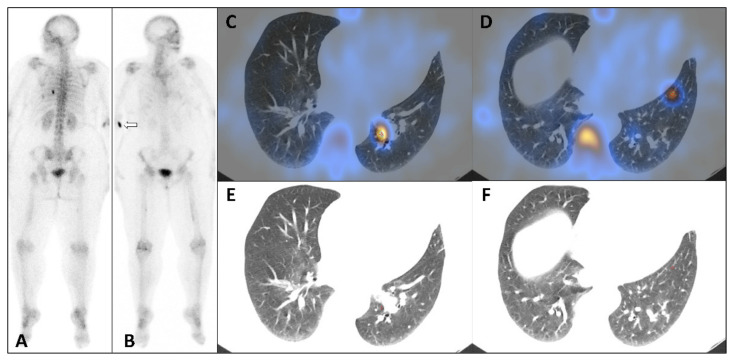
Whole body bone scan: posterior view (A), anterior view (B), axial SPECT-CT fused slices (C,D), correspondent CT slices (E,F). A 48-year-old woman was referred for a bone scan for assessment of bone metastasis from breast cancer. Posterior whole-body scan performed 2 h 30 min after IV injection of 825 MBq of ^99m^Tc-hydroxymethylene diphosphonate (^99m^Tc-HDP) highlighted two extra-osseous foci in projection of the 8th left rib (Figure 1A). Axial SPECT-CT fused slices showed an intense focal uptake in the left posterobasal lung segment close to proximal inferior lobe vessel (Figure 1C) and a less intense and more distal second focal uptake in the left anterobasal segment (Figure 1D). No abnormality was visible on correspondent CT slices (Figure 1E,F). Bone metastasis were limited to sternal body and left femoral diaphysis with mild osteoblastic activity. The patient had predominant liver metastasis, and carried out a combined treatment with trastuzumab, pertuzumab, letrozole, denosumab, calcium carbonate, and vitamin D3. The patient performed a contrast CT scan on the same day, thus, iobitridol was injected between ^99m^Tc-HDP intravenous injection and bone scan images acquisition. Fine helical slices showed no abnormality and especially no nodule or micronodule in the left lung. In order not to miss out a pulmonary embolism, ventilation/perfusion scintigraphy was performed five days later (Figure 2A,B), showing no significant perfusion defect. Eight months later, the follow-up bone scan showed no lung abnormality (Figure 2C,D), suggesting a non-pathological condition.
